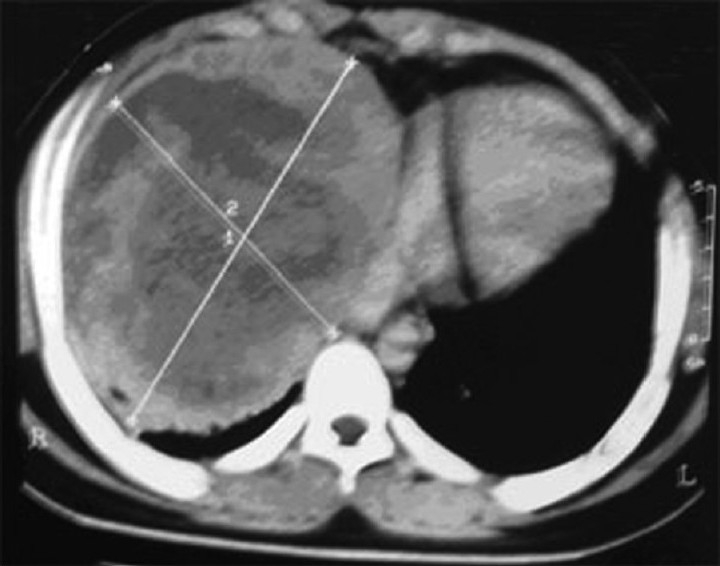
Computed tomography thorax showing inhomogeneously enhancing mass lesion involving the entire right hemithorax
